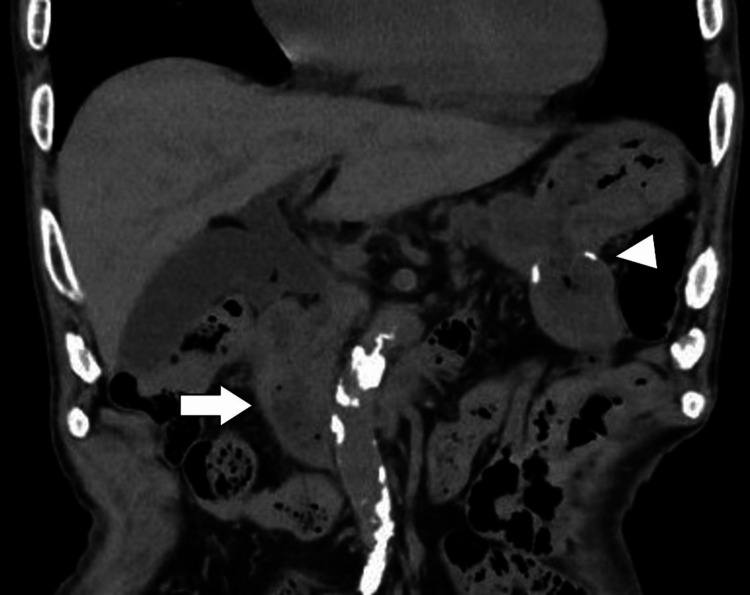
Abdominal computed tomography on day 77 Near-complete resolution of the intramural duodenal hematoma. The duodenum (arrow) and the gastrojejunostomy site (arrowhead) are indicated.
